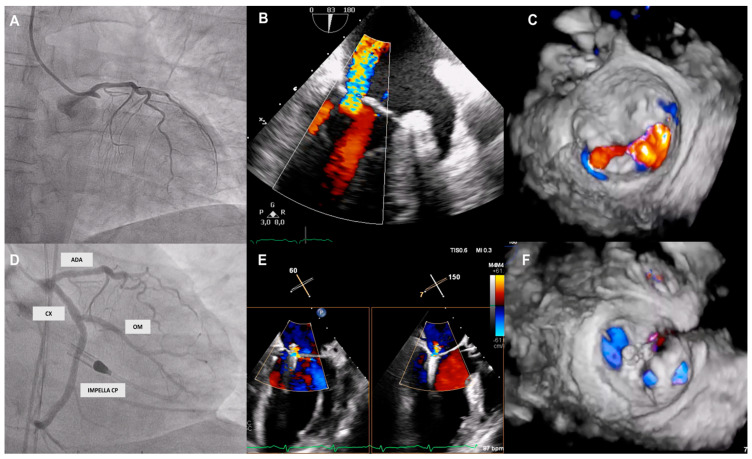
Multimodal imaging of a MitraClip implantation in a patient with IMPELLA CP^®^ device support. Angiography shows acute myocardial infarction due to occlusion of the proximal circumflex artery (A) with severe functional ischemic mitral regurgitation. On transoesophageal echocardiography (TEE) (B) and 3D reconstruction (C), a mid-level jet was observed, predominantly between P2-A2. (D). Angiography confirms the correct placement of IMPELLA CP^®^ device and the optimal results of the previously implanted stents. The valve was repaired by implantation of a MitraClip NTW device at A3-P3 and a second NT clip at A2-P2, with excellent results as assessed by TEE (E) and 3D reconstruction (F). ADA: anterior descendent artery; CX: circumflex; OM: obtuse marginal.
